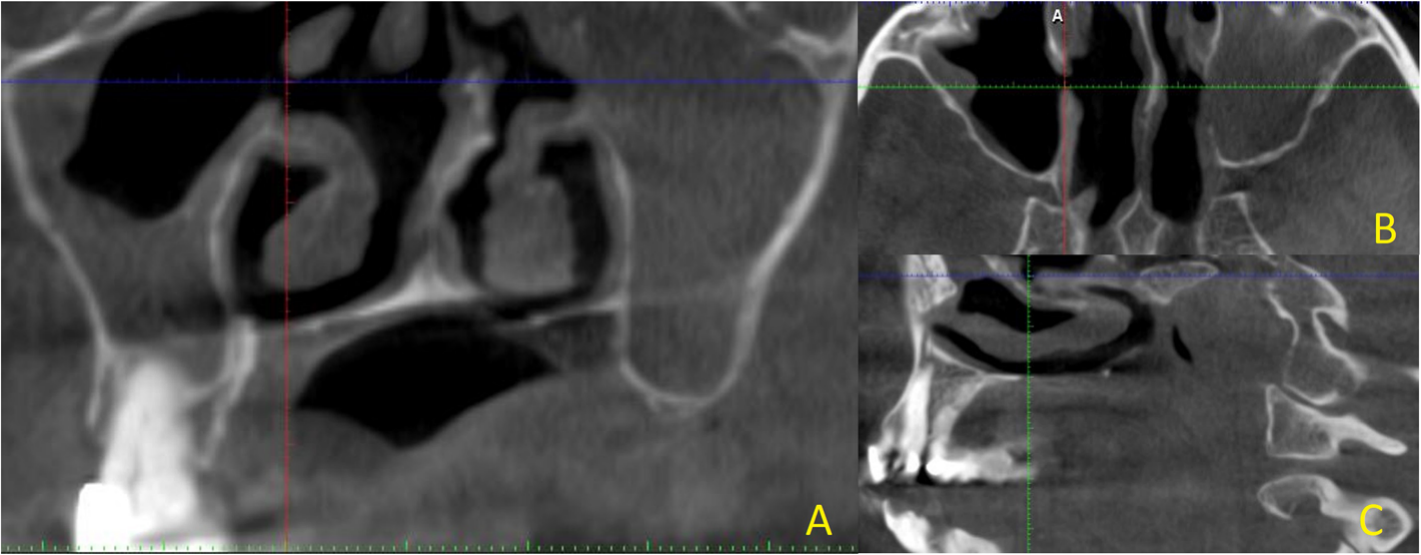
A coronal CBCT section showing AMO in the right maxillary sinus, deviation of the nasal septum and mucosal thickening in the floor of the sinus. B corresponding axial and C sagittal section. Coloured linear annotations are used to precisely locate the site of AMO in all three planes
